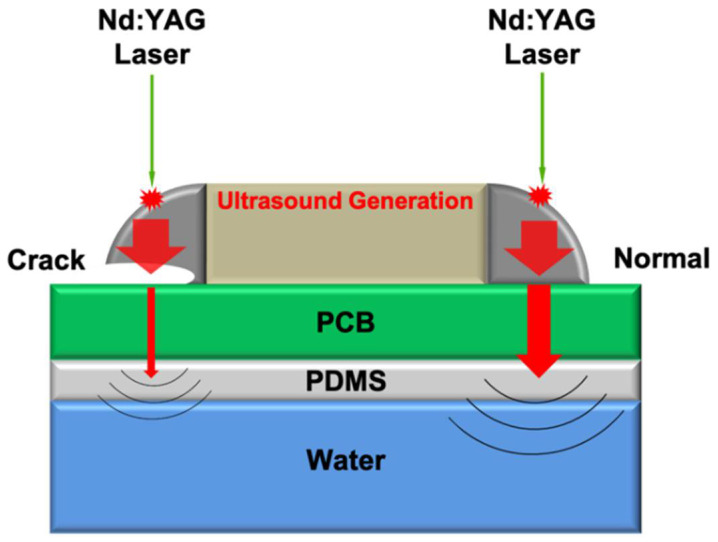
Principle of noncontact laser ultrasonic system.
